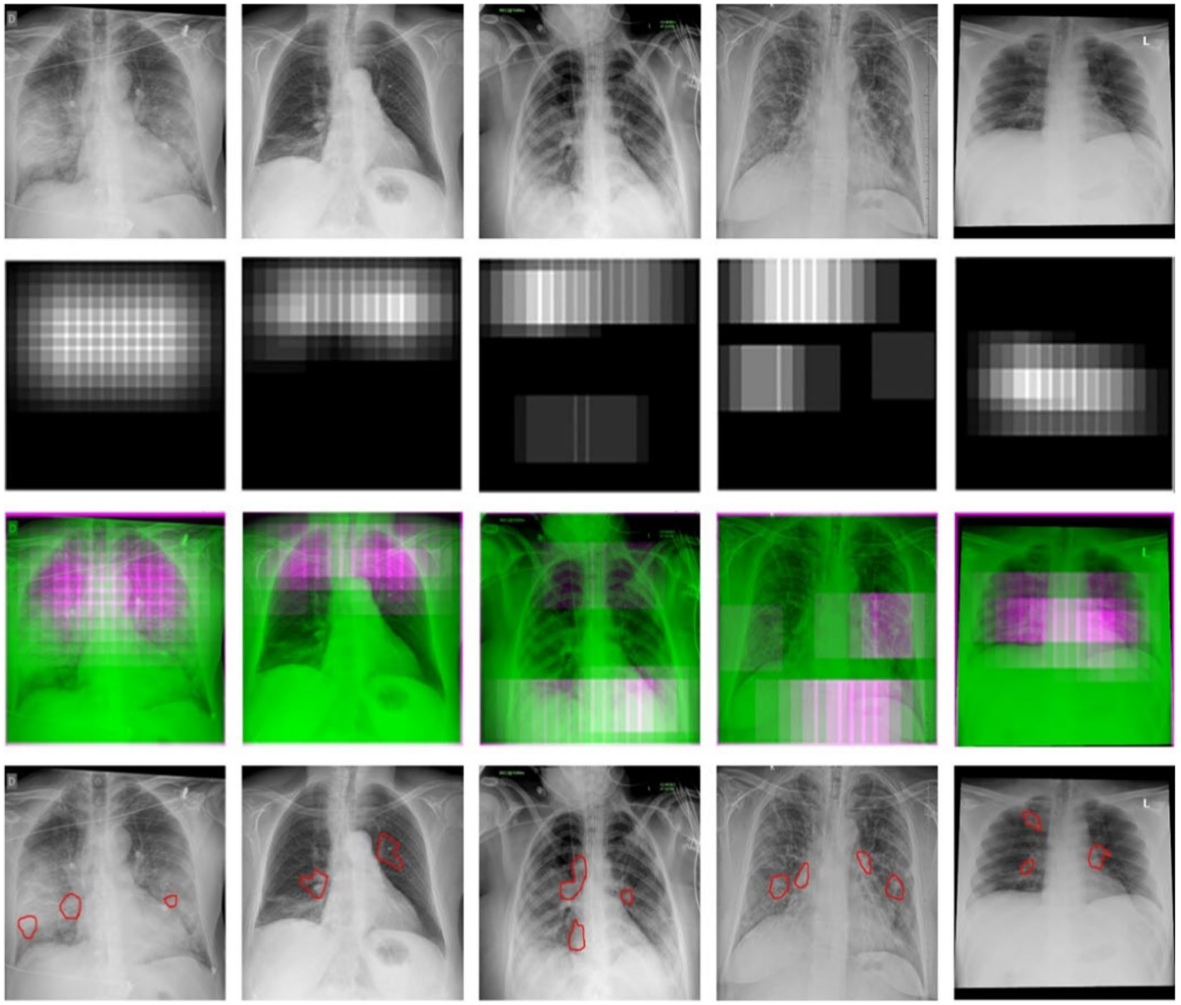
The detection of COVID-19-affected regions in the testing X-ray samples using the inception-v3 CNN model.
